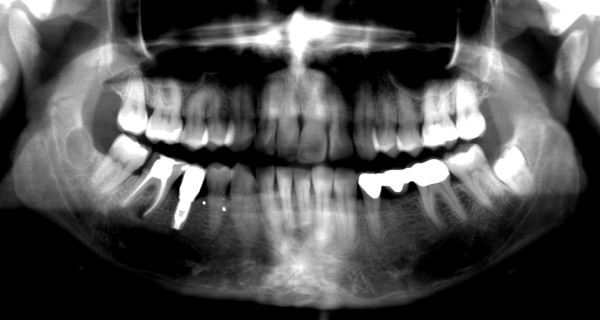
Panoramic radiograph showing the cyst-like radiolucency in the right mandibular third molar region. Seven years after removal of the right mandibular third molar.
